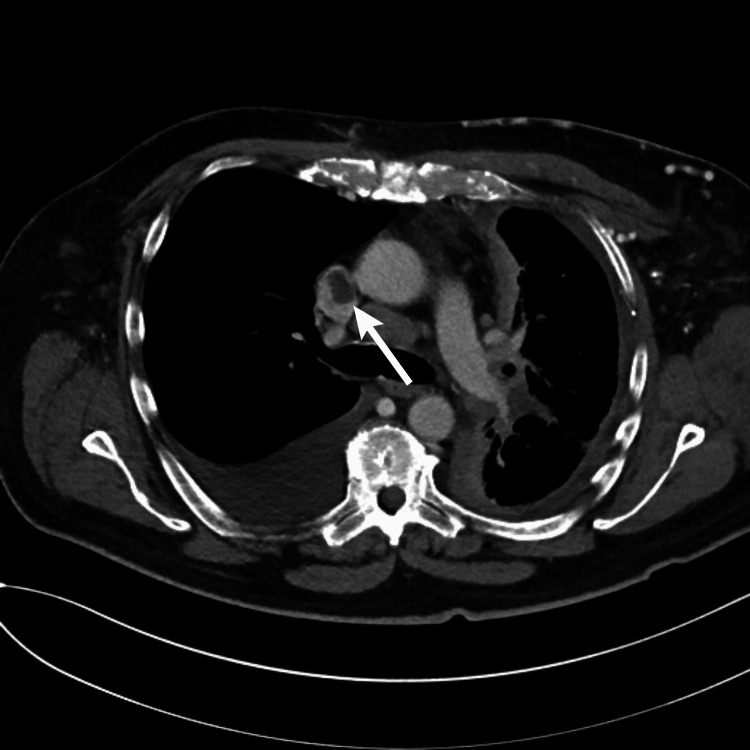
CT scan showing SVC thrombus marked with white arrow.
